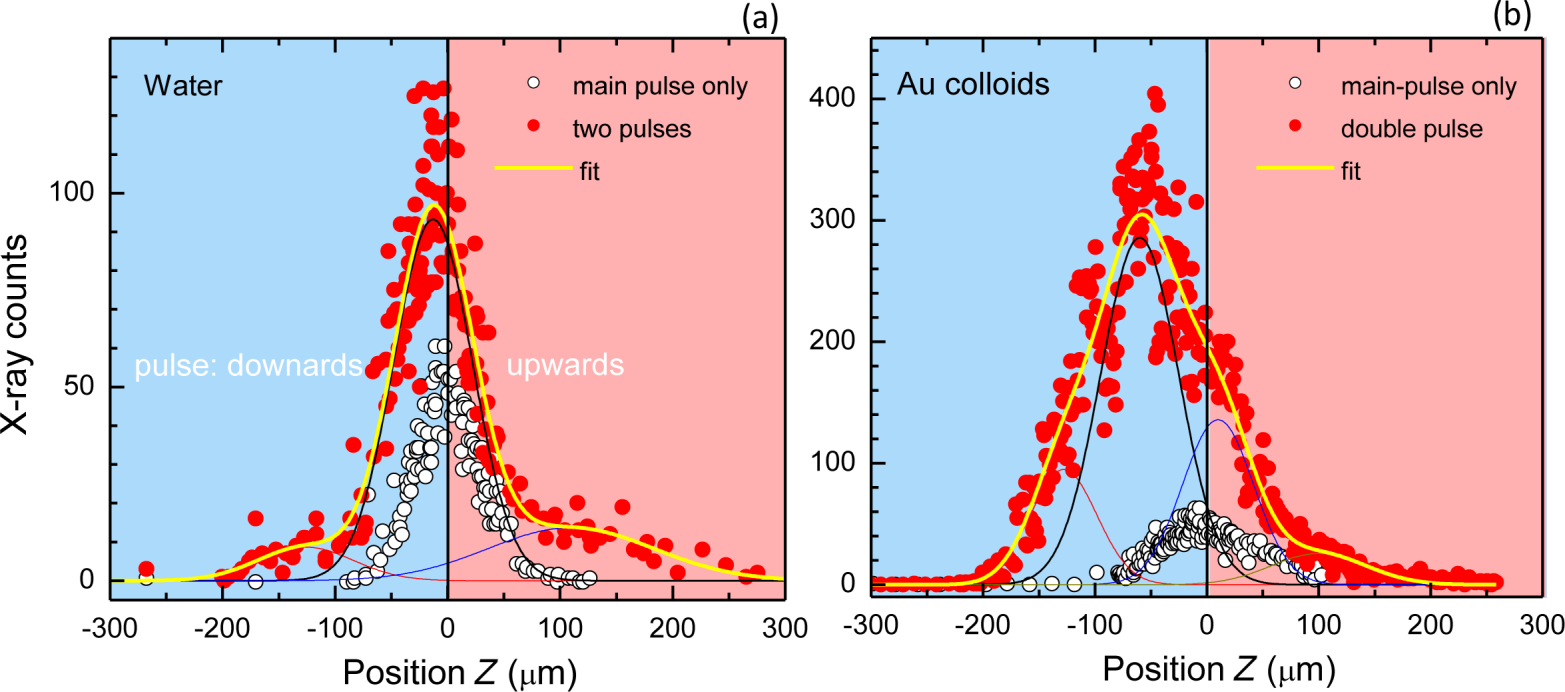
X-ray intensity at different positions of the water film (a) and the gold nanosphere colloidal solution film (b) at Δ*t* = 6 ns. The laser irradiates the solution film from the right side in these figures. The fit constitutes a multi-Gaussian convolution. The intensity of the pre-pulse is 80 μJ. Under double-pulsed excitation, the spatial profiles of X-ray intensity are significantly different in the cases of water and the gold nanospheres colloidal solution.
